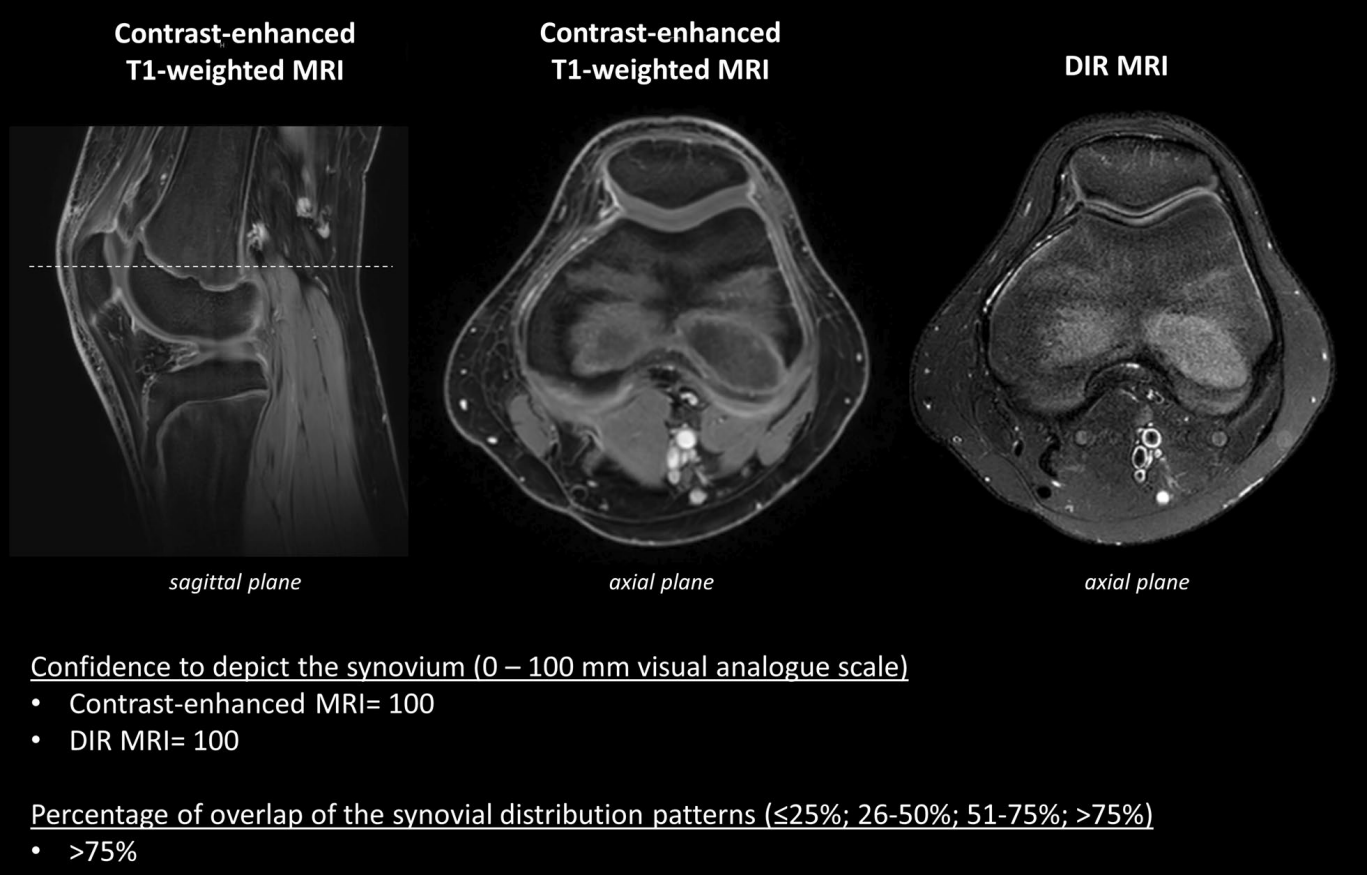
Images obtained in a 14-year-old boy, diagnosed with extended oligoarticular JIA, without knee arthritis. Confidence to depict the synovium was 100 (0–100 mm visual analogue scale) for the axial contrast-enhanced MRI and the axial DIR MRI. The percentage of overlap of synovial distribution patterns was scored as > 75%
